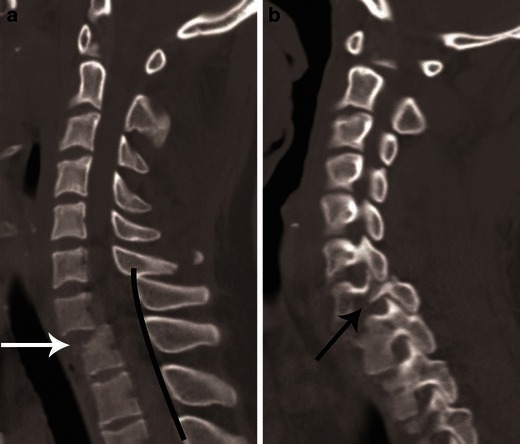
Hyperextension compression with traumatic spondylolisthesis. a Mid-sagittal CT image shows anterior listhesis of C7 over D1 (*white arrow*). This could result from hyperflexion distraction or, rarely, hyperextension injury. The spinolaminar line is continuous at the level of anterior translation, which suggests a hyperextension injury vector. b Left parasagittal image from the facet joint shows a vertically oriented fracture through the left pars interarticularis of C7 (*black arrow*) with impaction of the tip of the inferior articular process of C6 in the fracture gap—highly suggestive of hyperextension injury. Similar pars interarticularis fracture was also seen on the right side of C7 (not shown)
